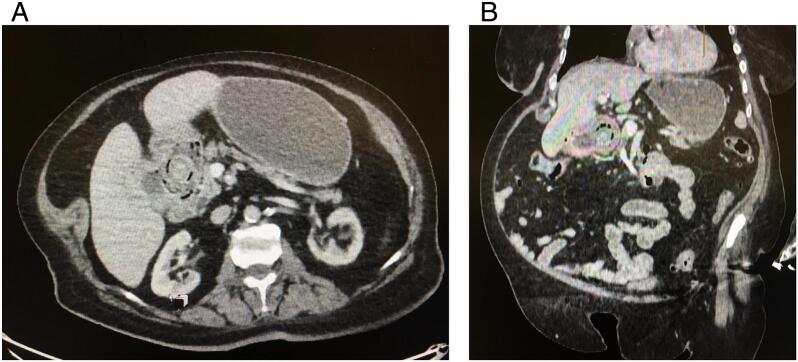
CT scan of the abdomen and pelvis showing artially-calcified gallstone(s) at the level of the superior duodenal flexure following cholecysto-duodenal fistulation causing upstream dilatation of the stomach suggestive of partial obstruction.
